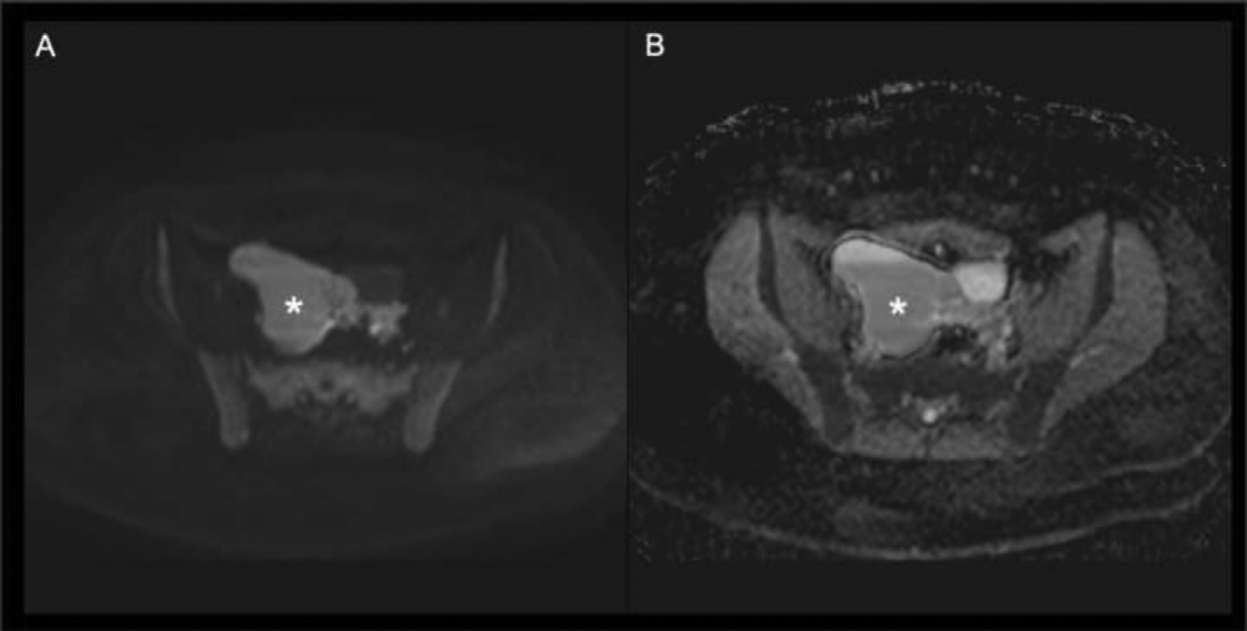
Axial high-b value (1000 sec/mm^2^) DWI- weighted image (A) and corresponding ADC map (B). Note diffusion restriction of the lesion (*) on DWI-weighted image due to the high viscosity of its content. On the ADC map a fluid-fluid level is clearly seen.
